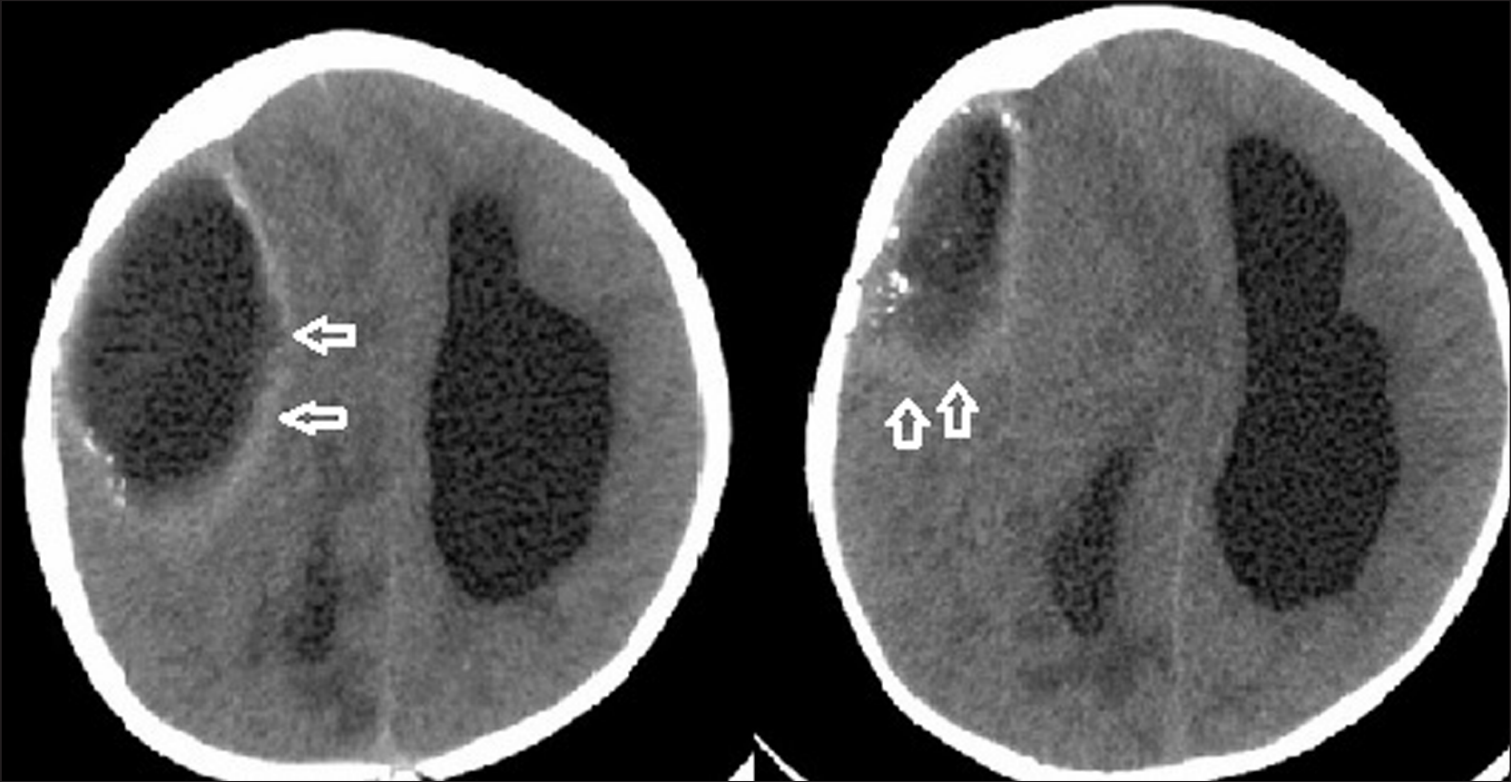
Noncontrast CT images detected a 4 cm right frontoparietal extraaxial collection with thick, calcified walls (arrows). Also, brain edema, hydrocephaly and subfalcine herniation were observed.
